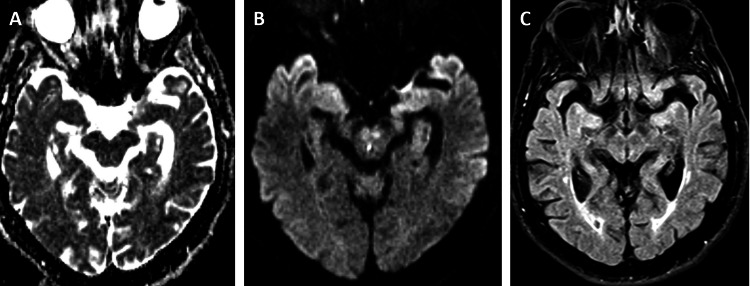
Case 2 MRI Axial MRI images including (A) ADC, (B) DWI, and (C) FLAIR.  Images demonstrate a small infarct in the right periaqueductal white matter along the anatomic course of the right MLF, without definite FLAIR correlate at this time. ADC, apparent diffusion coefficient; DWI, diffusion-weighted imaging; FLAIR, fluid-attenuated inversion recovery; MLF, medial longitudinal fasciculus.
